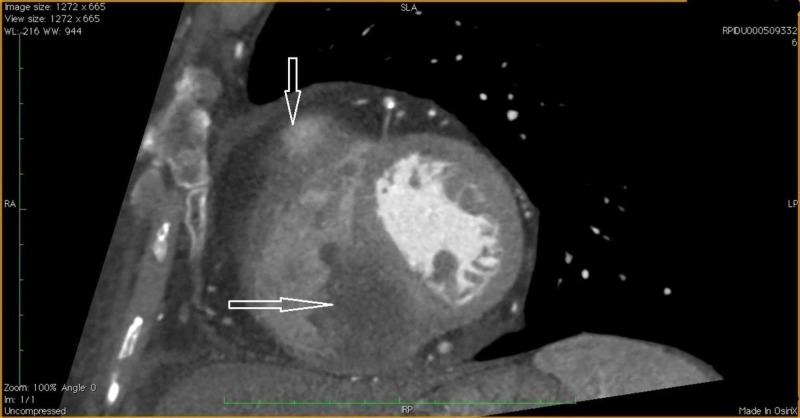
Computed tomography (CT) scan of the heart Multi planar reformation contrast-enhanced. Evidence of filling defect within the right ventricular cavity in correspondence with the interventricular septum.
